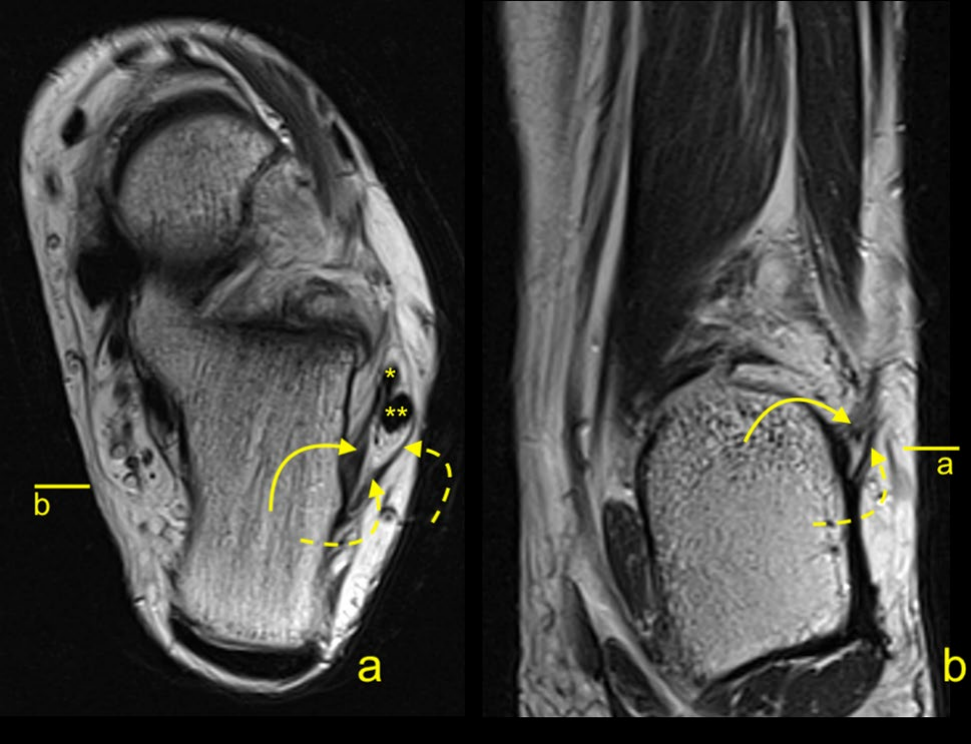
A 19-yeard-old patient with suspicion of the rheumatoid arthritis. No changes which correspond to this were found. The MRI did not reveal a tumor in the soft tissue. The communication between the CFL and the SPR. The axial section (a) and the corona section (b). Levels of cross-sections are labeled on both figures with lines and letters a and b. Connections of the CFL (curved arrow) with the superior peroneal retinaculum (dashed curved arrow), the peroneus brevis (*), and the peroneus longus ().
